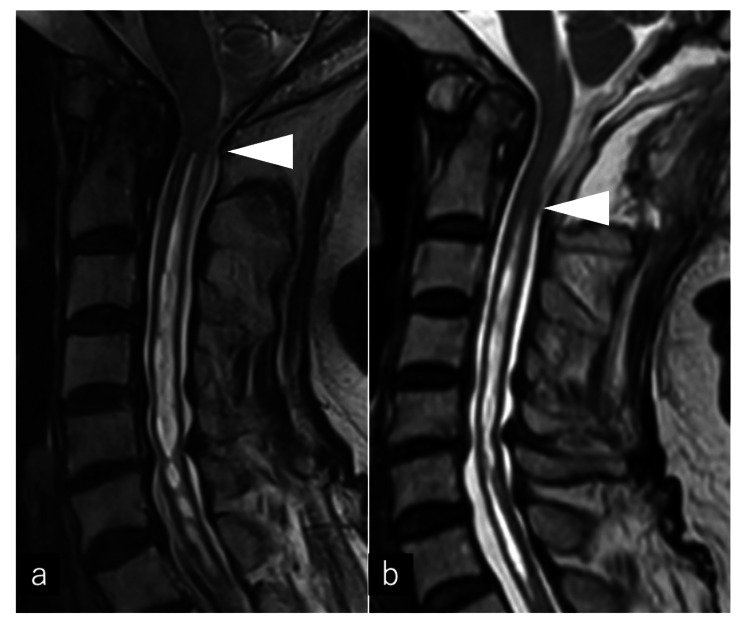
Comparison of preoperative and postoperative cervical spine magnetic resonance imaging (a) Preoperative sagittal T2-weighted image, (b) Postoperative sagittal T2-weighted image. The arrowhead indicates an intramedullary T2-hyperintense lesion representing a syrinx, which regressed postoperatively from the foramen magnum level to the C2 level.
